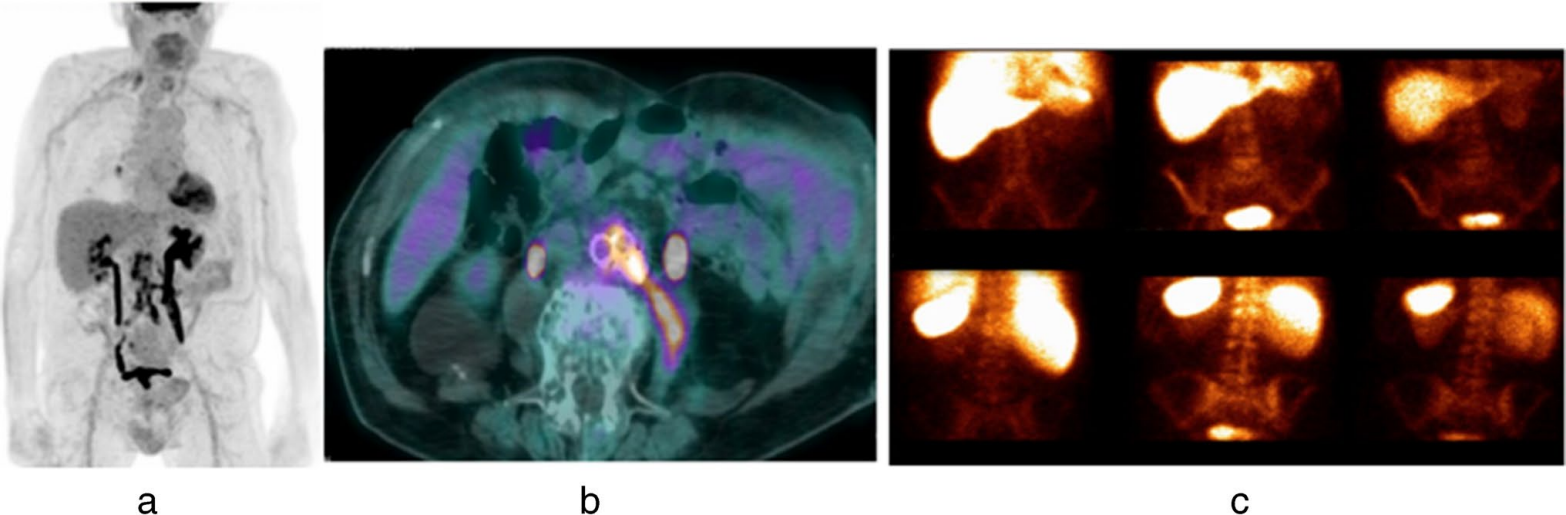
Example of TP at PET/CT and FN at ^99m^Tc-WBC scan. MIP images (a) and axial views of fused PET/CT images (b) were consistent with VGEI (score VI for the new scale and 5 for Sah scale) due to the presence of increased activity extended to peri-graft tissues (left psoas muscle). Planar antero-posterior views of ^99m^Tc-WBC scan (c) were negative. After NM examinations, *Candida tropicalis* was isolated in this patient
